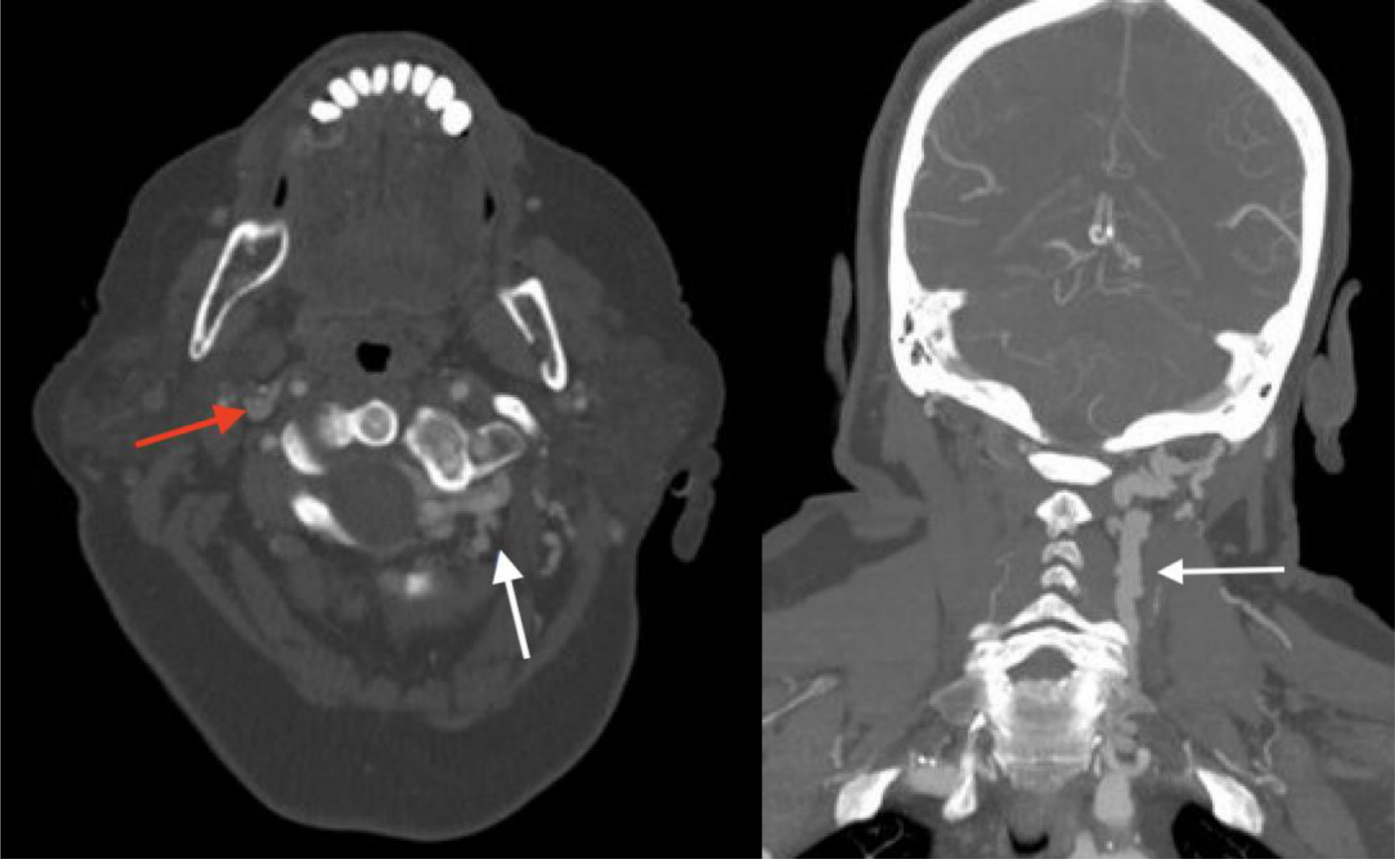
CTA axial (left), coronal (right): Prominence of left paraspinal veins (white arrow). Asymmetric and prominent vertebral venous drainage on the left initially concerning for an underlying vascular malformation. Red arrow demonstrates R internal jugular vein (IJV).
